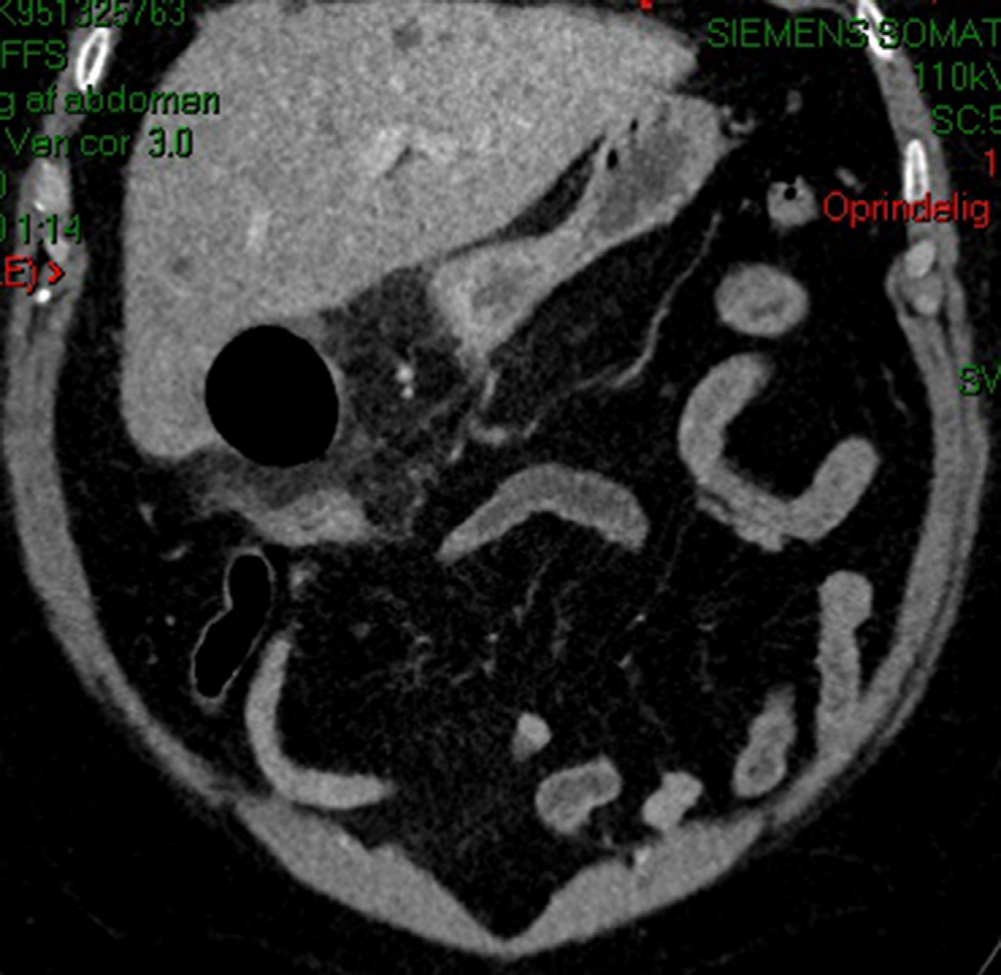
A contrast-enhanced CT scan coronal view showing acute emphysematous cholecystitis. Abnormally gas-filled gallbladder with inflammatory stranding of surrounding fat planes.
